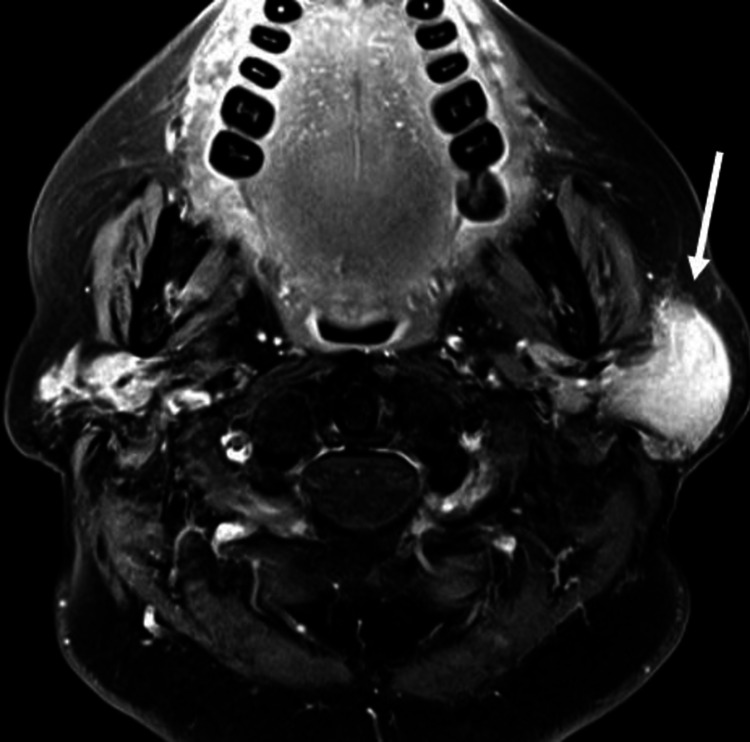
Axial fat-saturated post-contrast MRI at the level of the parotid glands showing homogeneous enhancement of left parotid space mass (white arrow). The irregular margins show infiltration into the deep parotid space at its medial aspect.
